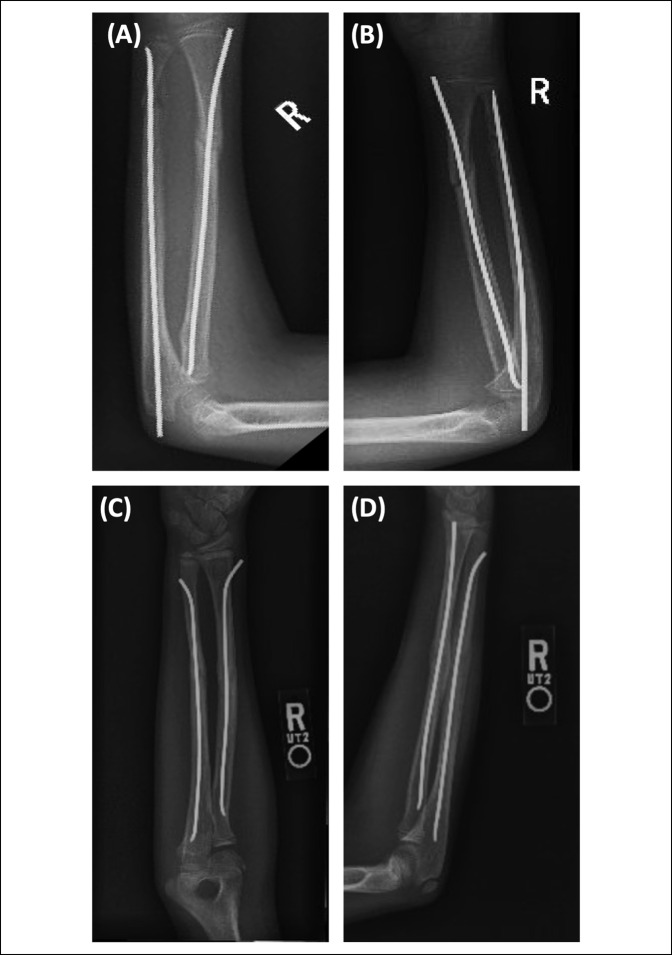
Radiograph of both-bone forearm fracture fixated with (A and B) antegrade intramedullary nailing of the ulna and retrograde nailing of the radius and (C and D) retrograde intramedullary nailing of both the ulna and radius in a skeletally immature patient.
